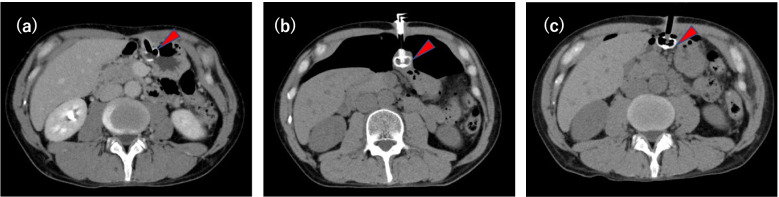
Computed tomographic images. Preoperative computed tomographic scans (a) showed percutaneous endoscopic gastrostomy bumper (red arrow) fixing stomach properly. Postoperative computed tomographic scans (b) showed percutaneous endoscopic gastrostomy bumper (red arrow) dislodged from abdominal wall. Postoperative day 4 computed tomographic scans (c) showed percutaneous endoscopic gastrostomy bumper (red arrow) protrude from stomach
